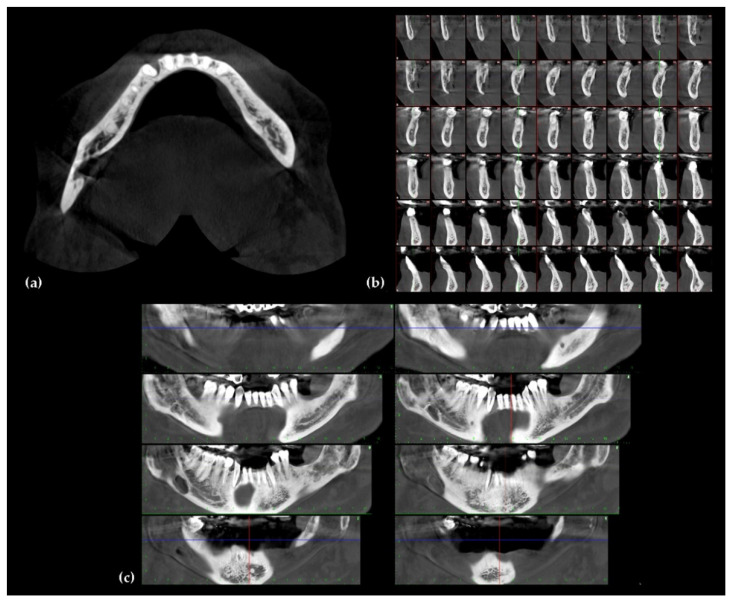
A cone beam computed tomography (CBCT) multiplanar reconstruction of lower jaw: (a) axial view, (b) sagittal view, and (c) coronal view.
